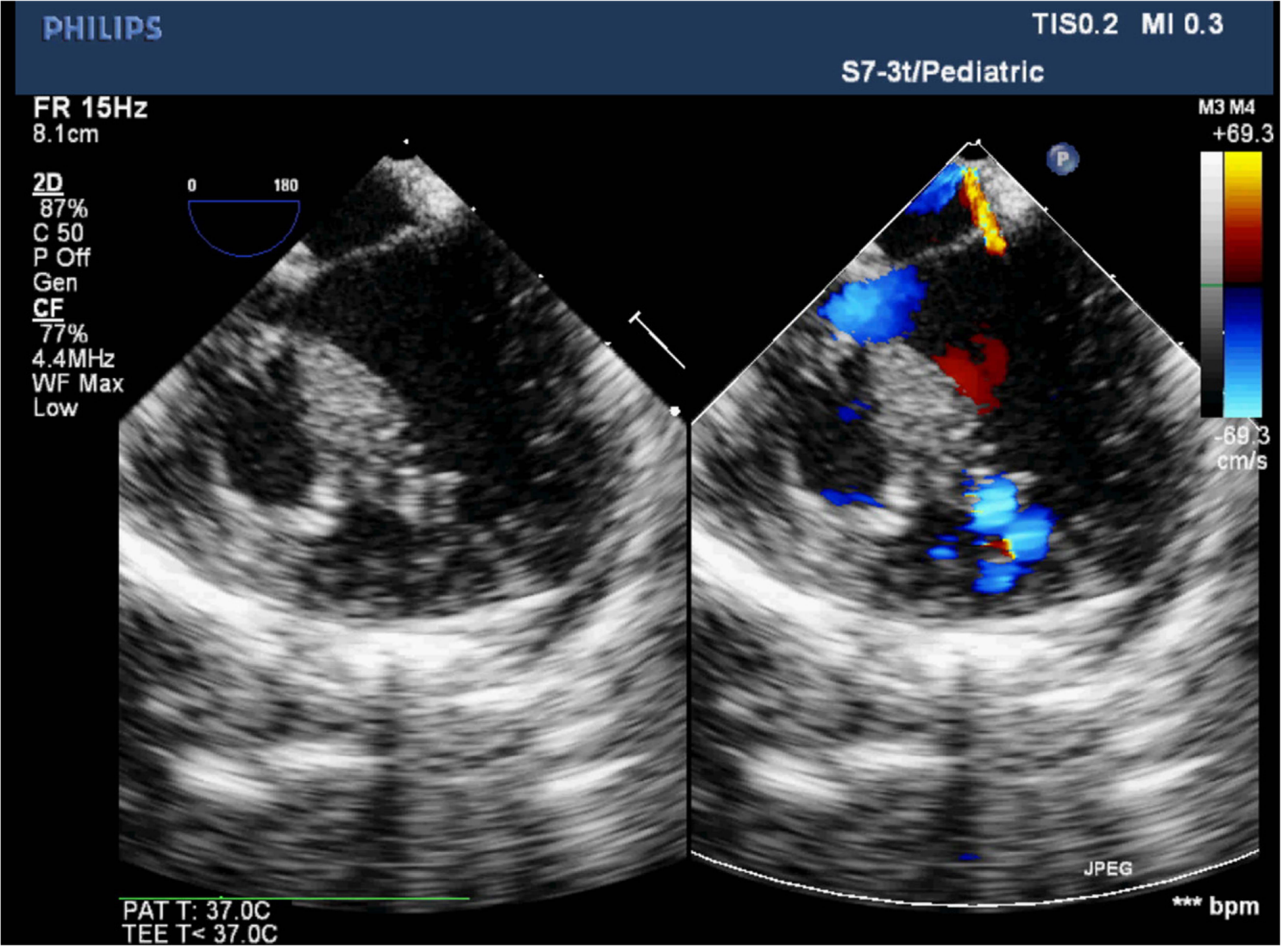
Transesophageal echocardiography (four chamber view) picture showing the apical portion of the right ventricle is excluded from the right ventricle and all the left-to right ventricular shunts are obliterated, although there are still blood flows inside the interventricular septum
